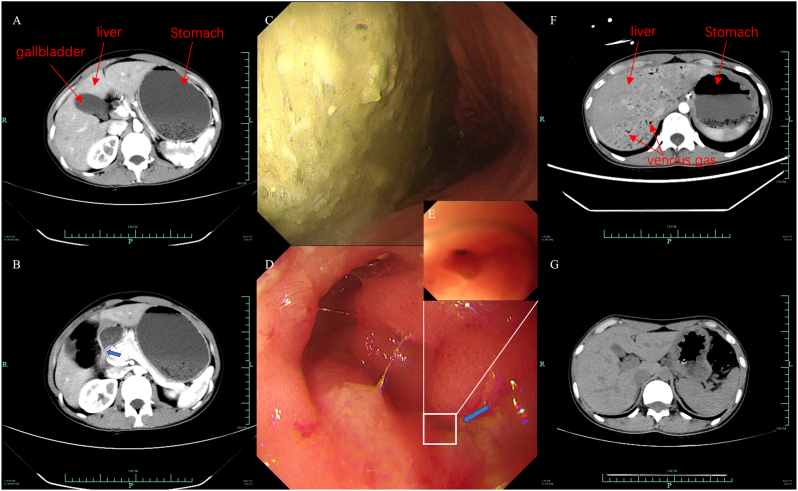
Imaging and endoscopic features of duodenal bulb stenosis with secondary gastric dilatation and transient portal venous gas. (A) Contrast-enhanced CT shows massive gastric dilatation. (B) CT reveals a tight, fibrotic stenosis at the duodenal bulb. (C) Gastroscopy demonstrates a markedly distended stomach filled with retained chyme. (D) The duodenal bulb appears distorted on endoscopy. The area indicated by the blue arrow is the narrow part. (E) A critically narrowed lumen (<1 mm) prevents passage of an ultrathin gastroscope. (F) Emergency CT shows extensive portal venous gas and submucosal air in the gastric wall without free air. (G) Follow-up CT two days later shows complete resolution of portal venous gas with only mild gastric wall edema.
